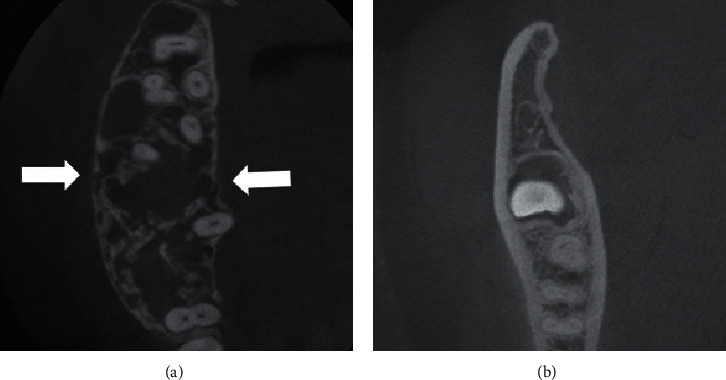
Axial CBCT images. (a) Maxillary odontogenic myxoma (Patient 3) at the dentate area, including more than 3 teeth with bone expansion and perforation (arrows). (b) Mandibular odontogenic myxoma (Patient 9) with unerupted third molar without bone perforation.
